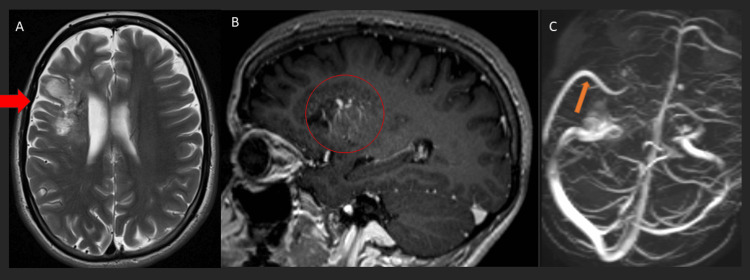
Brain MRI of the patient in the context of a developmental venous anomaly at the one-year follow-up. The T2 weighted axial image (A) showed significant regression of the vasogenic edema in the right frontal lobe with encephalomalacia and gliosis. However, the post-gadolinium T1 axial image (B) and MR venogram 3D image (C) showed persistent medullary draining veins and a large collector vein draining into the right transverse sinus, representing the developmental venous anomaly.
